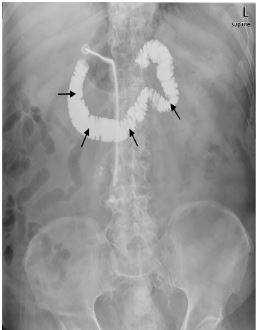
Contrast-enhanced abdominal radiography outlining the duodenum/proximal jejunum (arrows).
